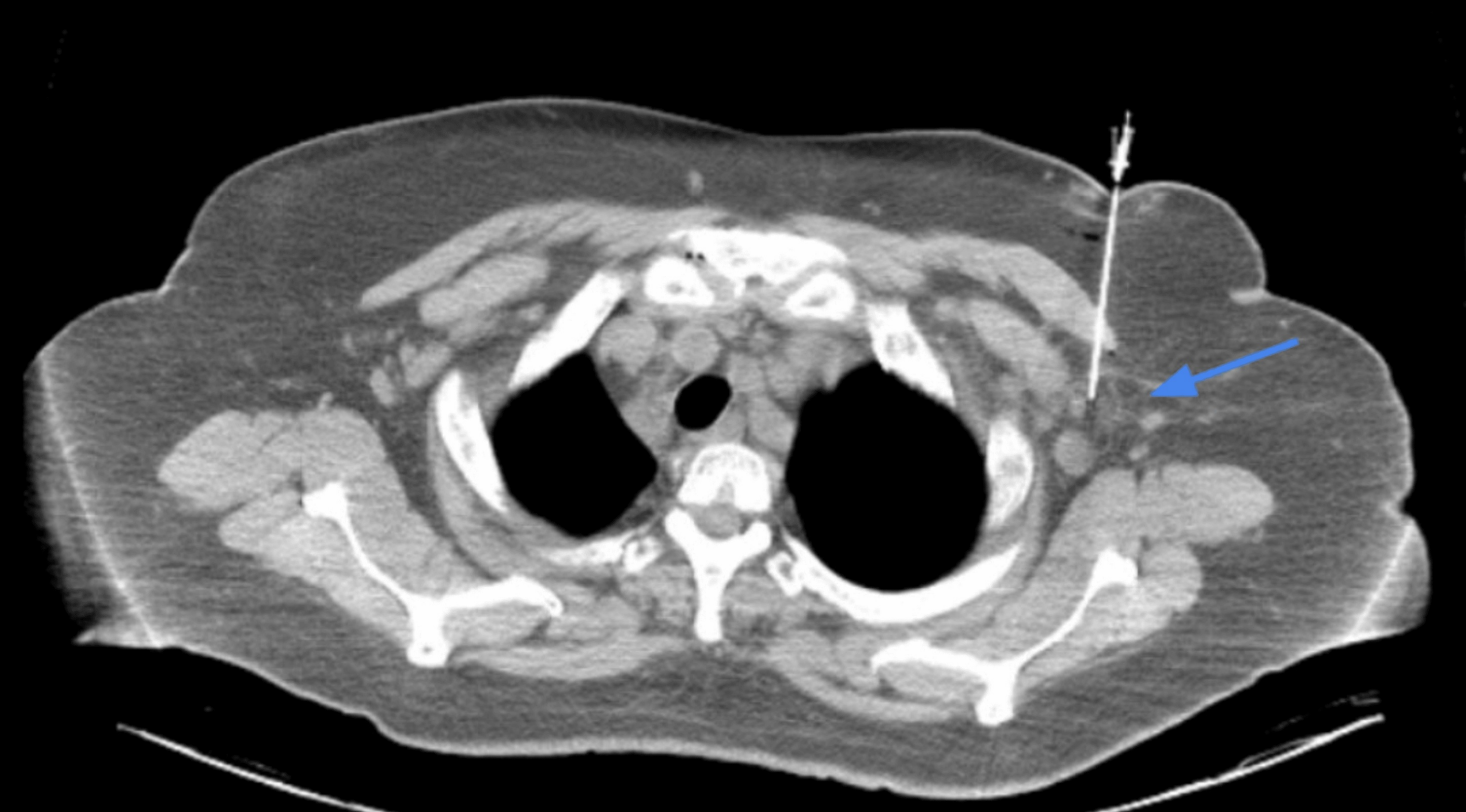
CT-guided core biopsy of a left axillary 2 cm lymph node (blue arrow).
